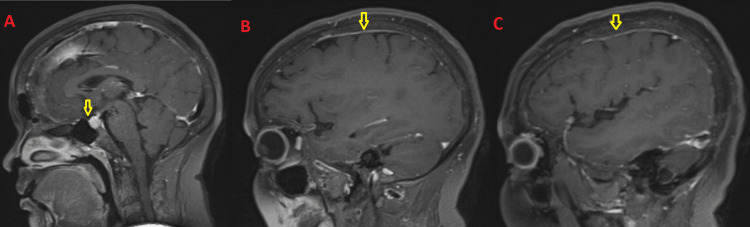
MRI scan of the brain. A: The pituitary is mildly bulky (10 mm) with a mildly thickened enhancing stalk (arrows). B, C: There is smooth enhancing pachymeningeal thickening along the bilateral cerebral convexities (arrows).
